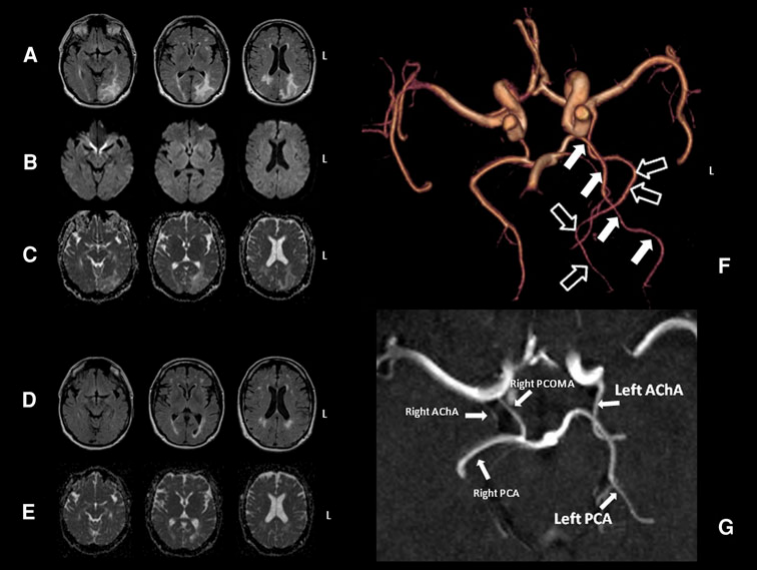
The first MRI (a–c) showed cortical–subcortical patchy hyperintense areas on T2 FLAIR-weighted images (a) localized in the parietal–occipital lobes with asymmetric representation for left predominance. No signal alteration was seen in DWI images (b) in presence of high signal on ADC maps indicating vasogenic edema (c). MRI exam after 3 months demonstrated complete resolution of the hyperintense areas on FLAIR-weighted (d) images and ADC maps (e). The MR-angiography of intracranic circle, performed with the 3D-TOF technique (f, g), showed the presence of left hyperplastic AChA (*white arrows*, tridimensional images f) supplying partially the distribution of the ipsilateral posterior cerebral artery (*PCA*) (*arrows*, tridimensional images f). In g the same anatomical abnormalities is represented by MIP reconstruction
